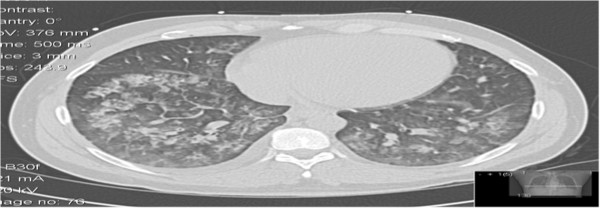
Computed tomography of the chest without contrast - bilateral peribronchovascular airspace consolidation, ground-glass opacity with interlobular septal thickening.
